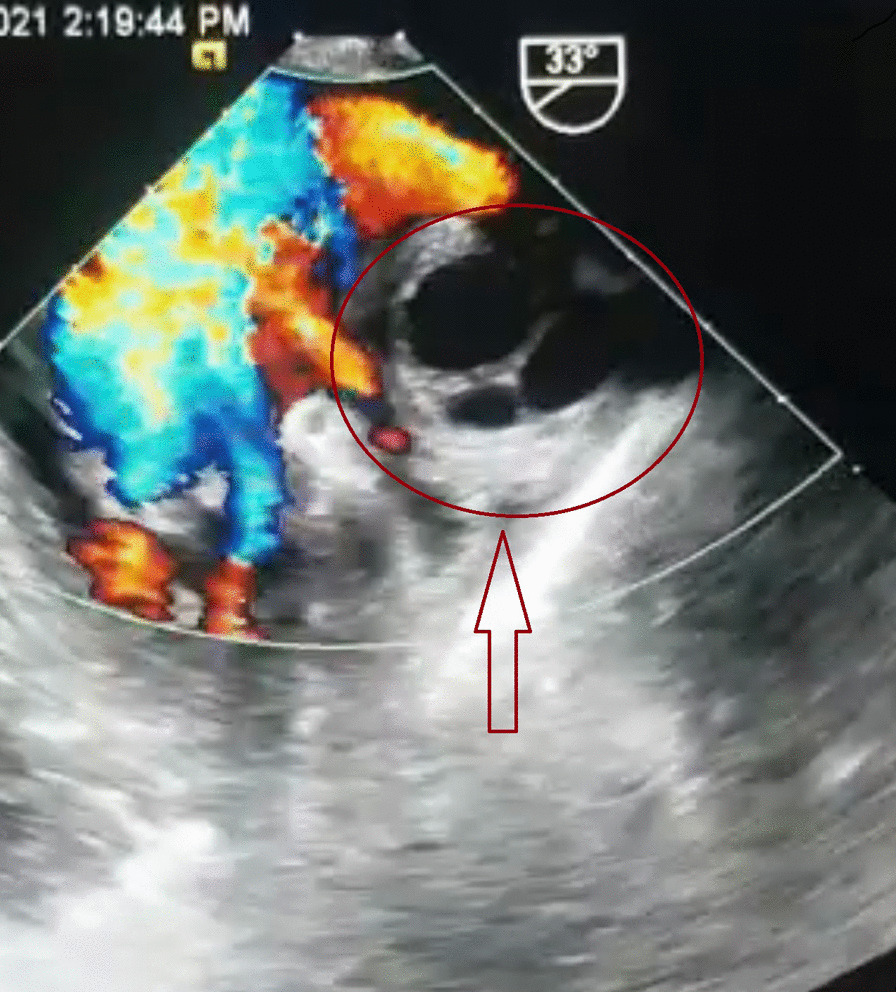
A Gharbi type II hydatid mass on transesophageal ultrasound
